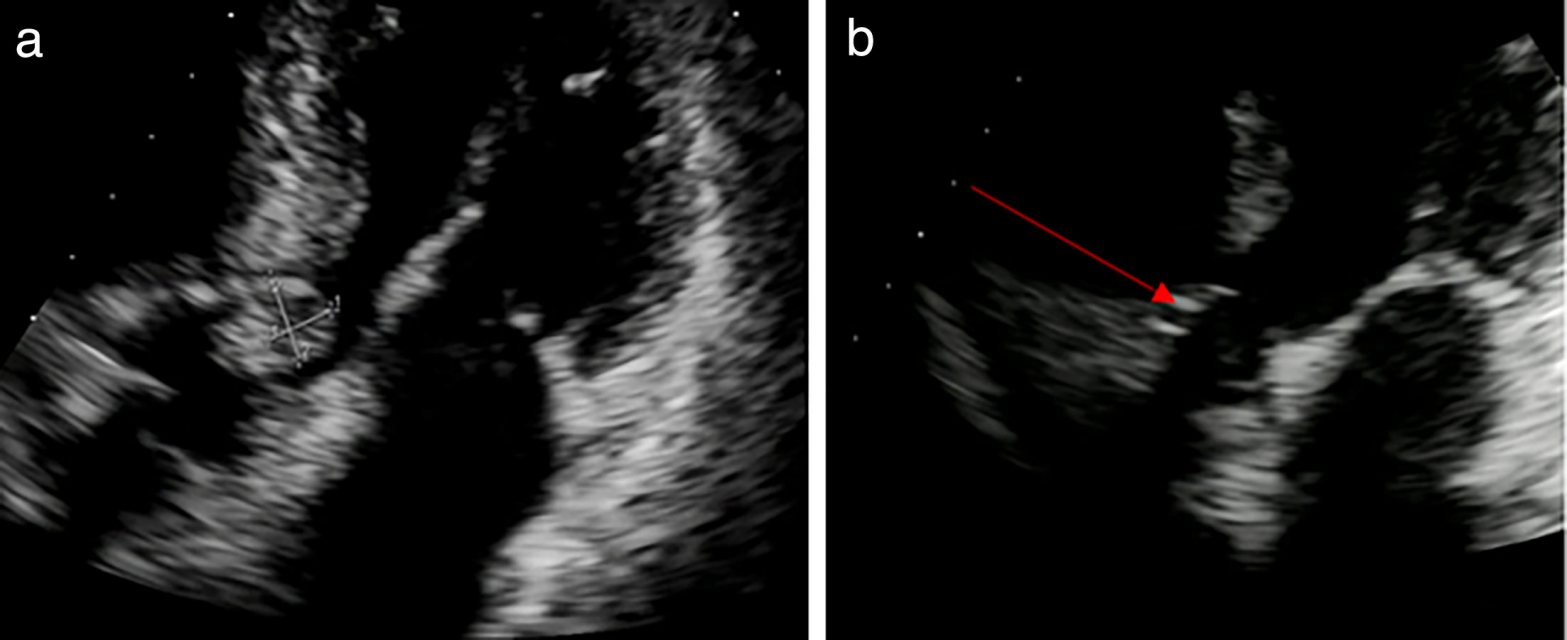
(a) Patient 2, transthoracic echocardiogram pre-treatment. A 1 × 1.2 cm echogenic mass on the aortic valve with associated moderate aortic stenosis and aortic regurgitation in keeping with a vegetation. (b) Patient 2, transthoracic echocardiogram post-treatment. Well-seated aortic valve bio-prosthesis with no regurgitation (arrow).
